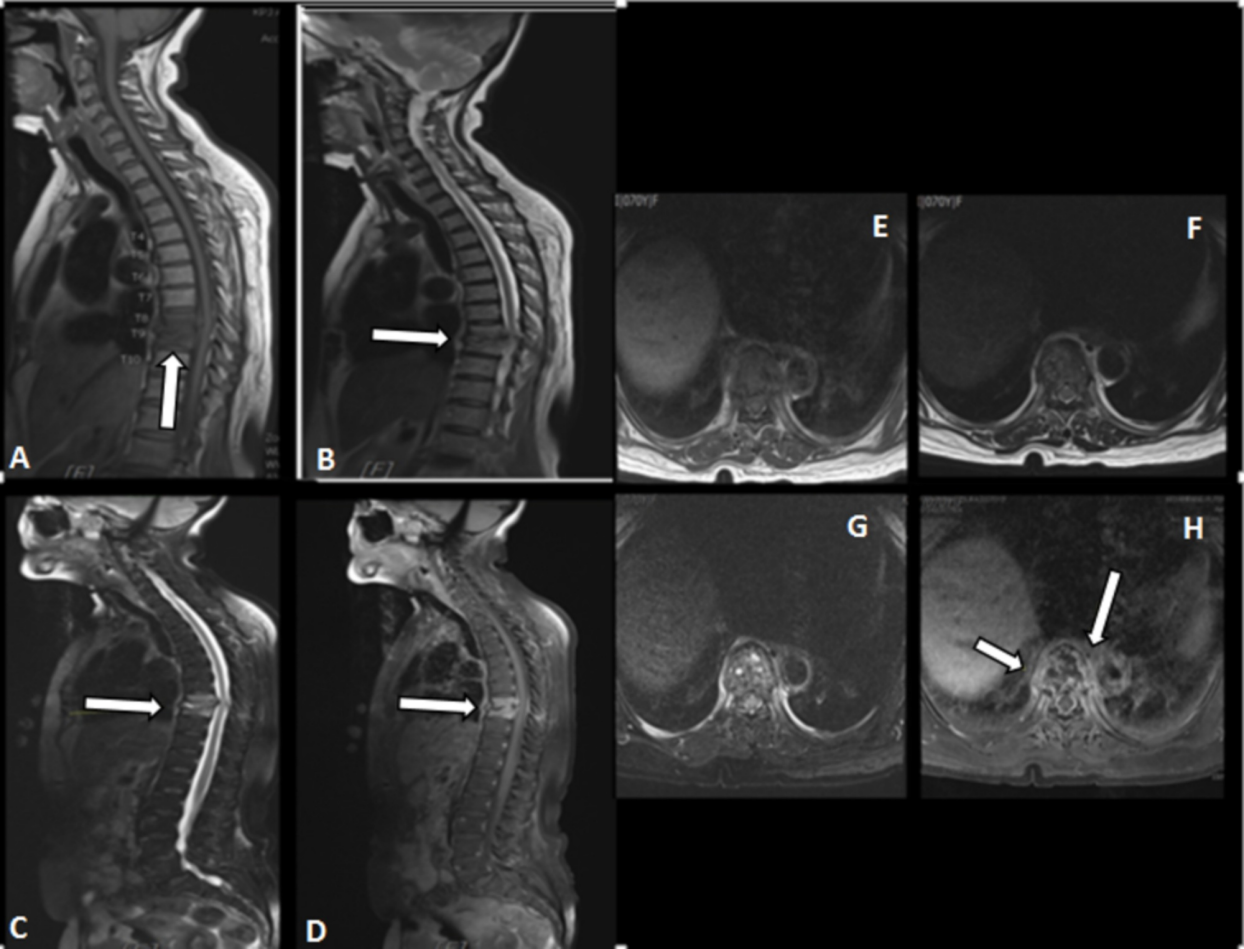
MRI films of the spine at the two months follow-up, suggestive of spondylodiscitis of T8 and T9 with suspicion of tuberculosis infection A-D : sagittal view; E-H : axial view
